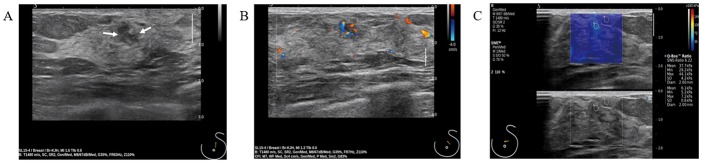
Benign intraductal papilloma diagnosed at ultrasound-guided core needle biopsy. (A-C) (A) B-mode ultrasound shows an irregular shaped hypoechoic mass (1 cm, arrows). (B) Color Doppler showing increased vascularity in the mass. This mass was assessed as category 4B. (C) Shear-wave elastography image showing a light blue color (Emax, 44.1 kPa). This mass was finally surgically excised and proved as benign intraductal papilloma.
